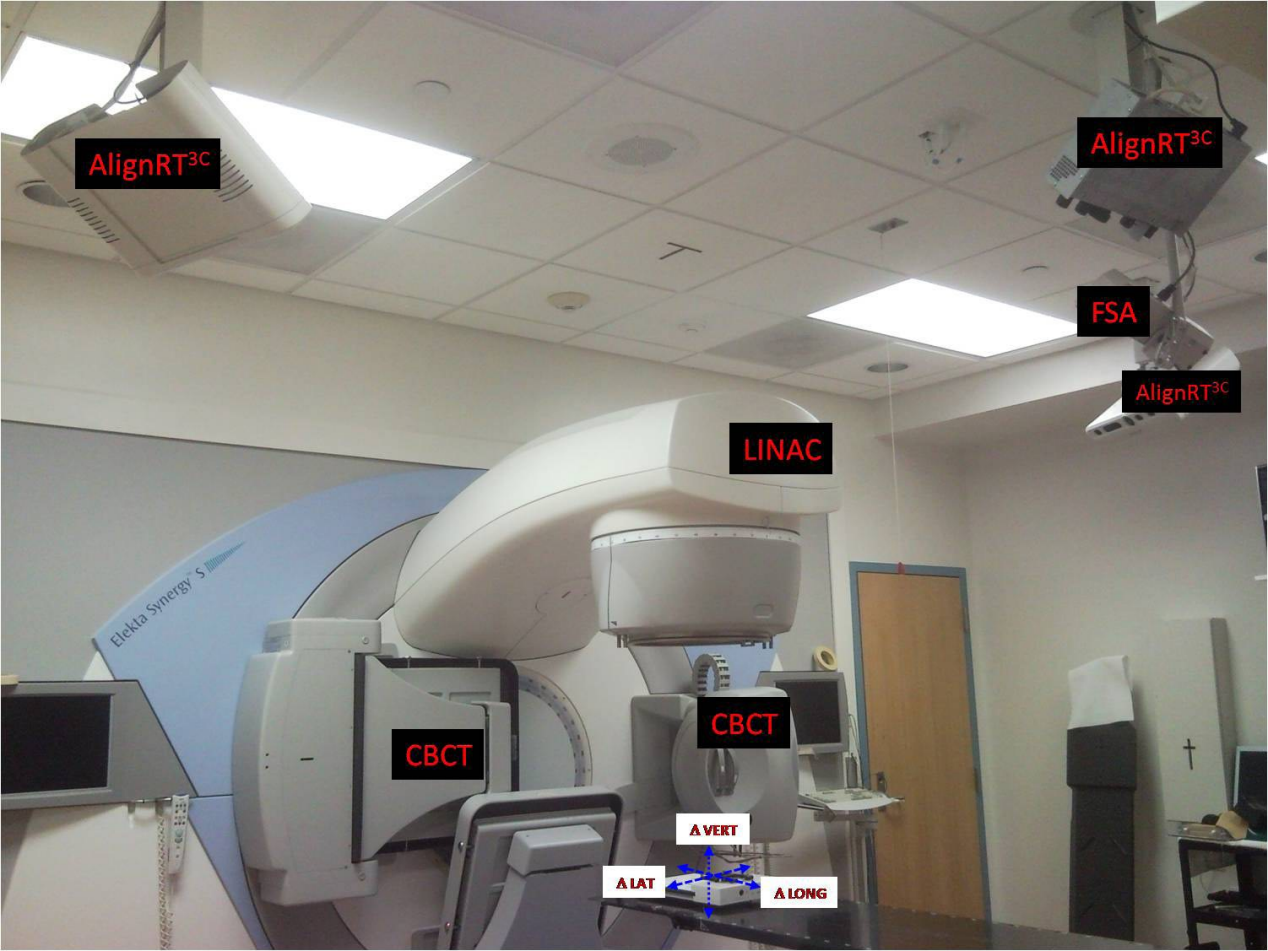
Photograph of the Linac room with three IGSPSs: CBCT, FSA, and ${\text{AlignRT}}^{3C}$. The arrows indicate the three translational and rotational directions: Δ LAT = lateral (left–right), Δ LONG = longitudinal (superior–inferior), and Δ VERT = vertical (anterior–posterior).
